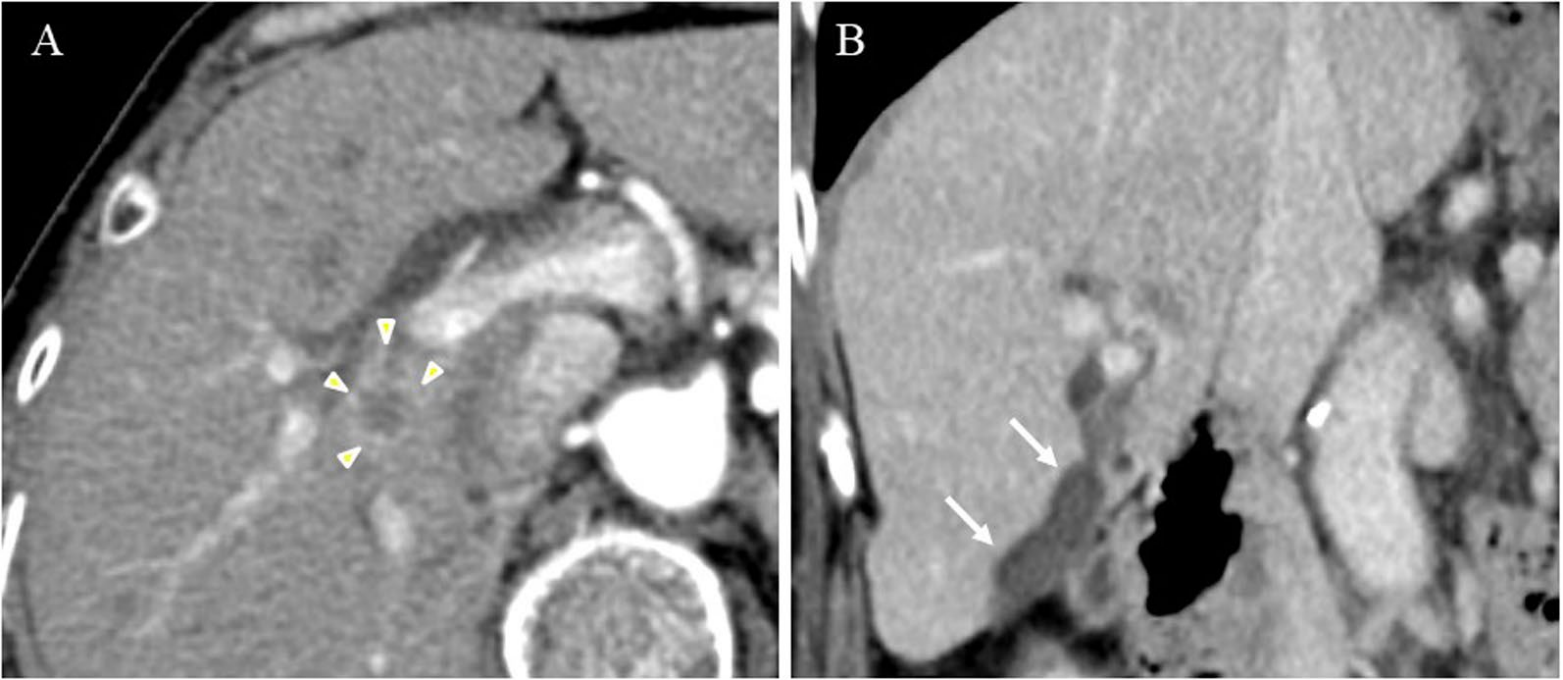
Preoperative contrast-enhanced CT findings. A In the axial view of the arterial phase, a 15 mm irregular circumferential ring-enhancing mass lesion was observed on the dorsal side of the right hepatic hilum (arrowheads). B In the coronal view of the venous phase, a cystic dilatation of the bile duct measuring 7 mm in diameter was observed in segment 6 bile ducts (B6) (arrows)
